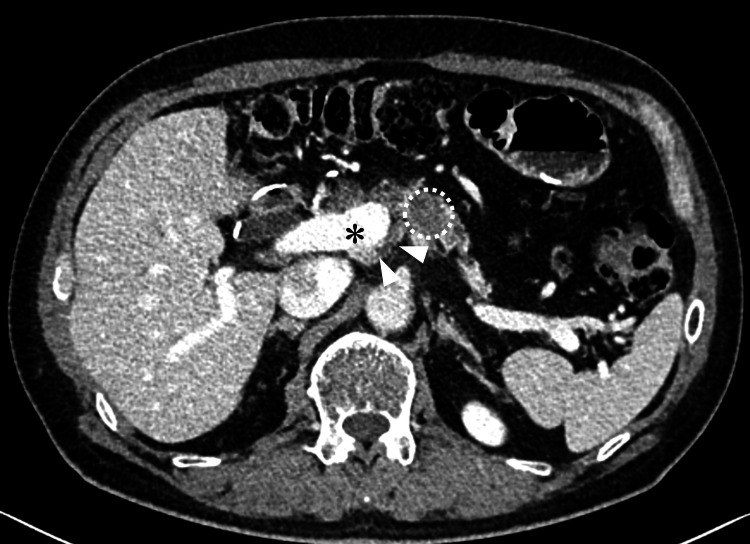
The contrast-enhanced computed tomography image after neoadjuvant chemotherapy Arrowheads indicate the dorsal pancreatic parenchyma; the asterisk indicates the portal vein; dotted lines outline the pancreatic tumor.
